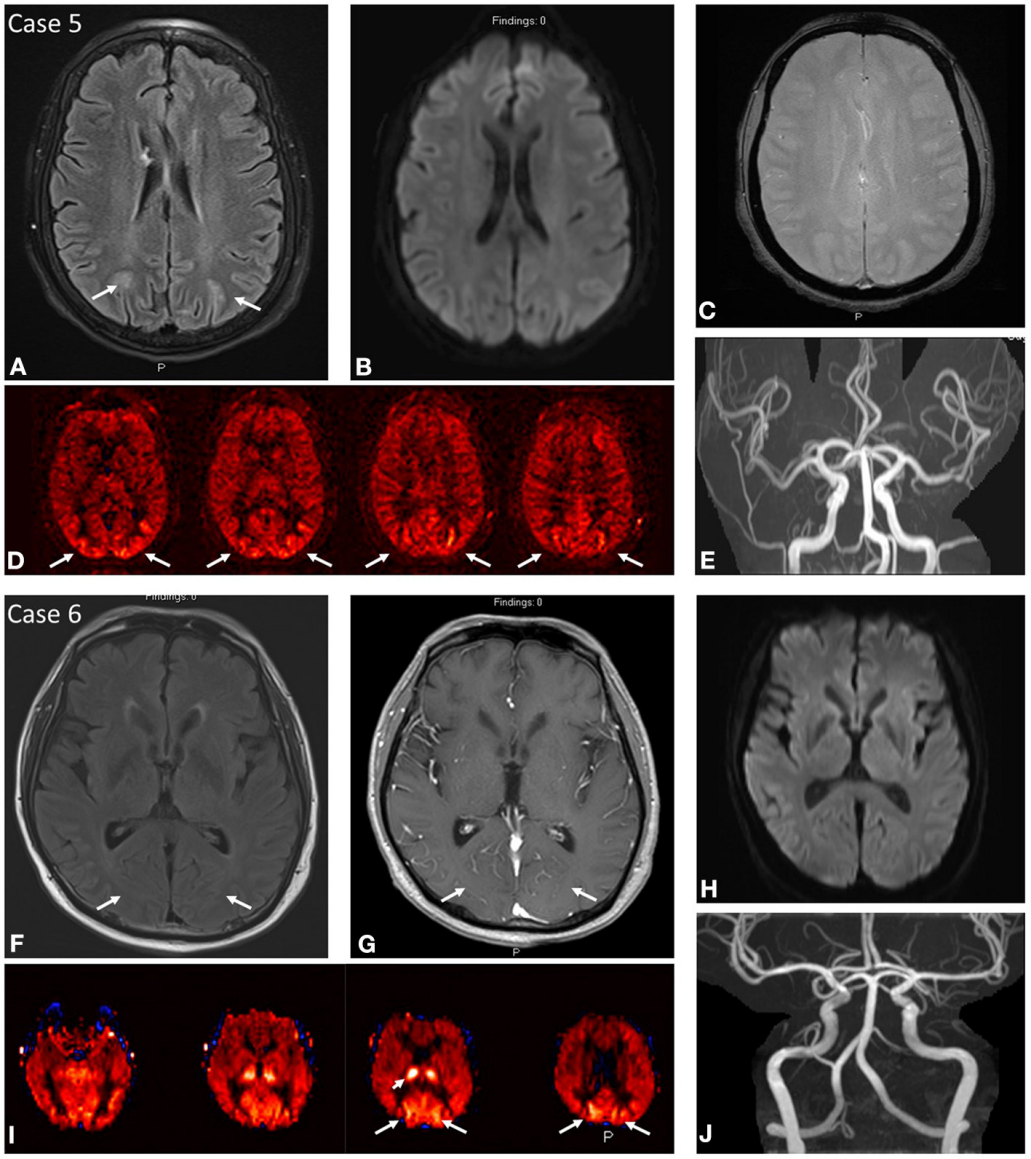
Case 5: A 64-year-old female presenting with uncontrolled hypertension of 205/93 and past medical history notable for sickle cell anemia. MRI brain at initial presentation demonstrates abnormal FLAIR hyperintensity (A) in the subcortical white matter of the bilateral parieto-occipital regions with corresponding regions of ASL signal hyperintensity (D). No DWI (B) or SWI (C) signal abnormality. No focal arterial high-grade stenosis or occlusion on MR angiography (E). Case 6: A 61-year-old male presenting with acute unresponsiveness in setting of prolonged hospital course due to COVID-19 pneumonia/ARDS with refractory hypoxemia and hypercarbia complicated by cardiac arrest, gastrointestinal bleeding, hypotension on pressors, and eventual bilateral lung transplant on tacrolimus. MRI brain demonstrates ASL signal hyperintensity (I) in the bilateral occipital lobes with asymmetrically increased signal in the right thalamus. Findings correspond to subtle FLAIR hyperintensity (F) with mild vascular congestion on post-contrast imaging (G). No DWI (H) signal abnormality. No focal arterial high-grade stenosis or occlusion on MR angiography (J).
